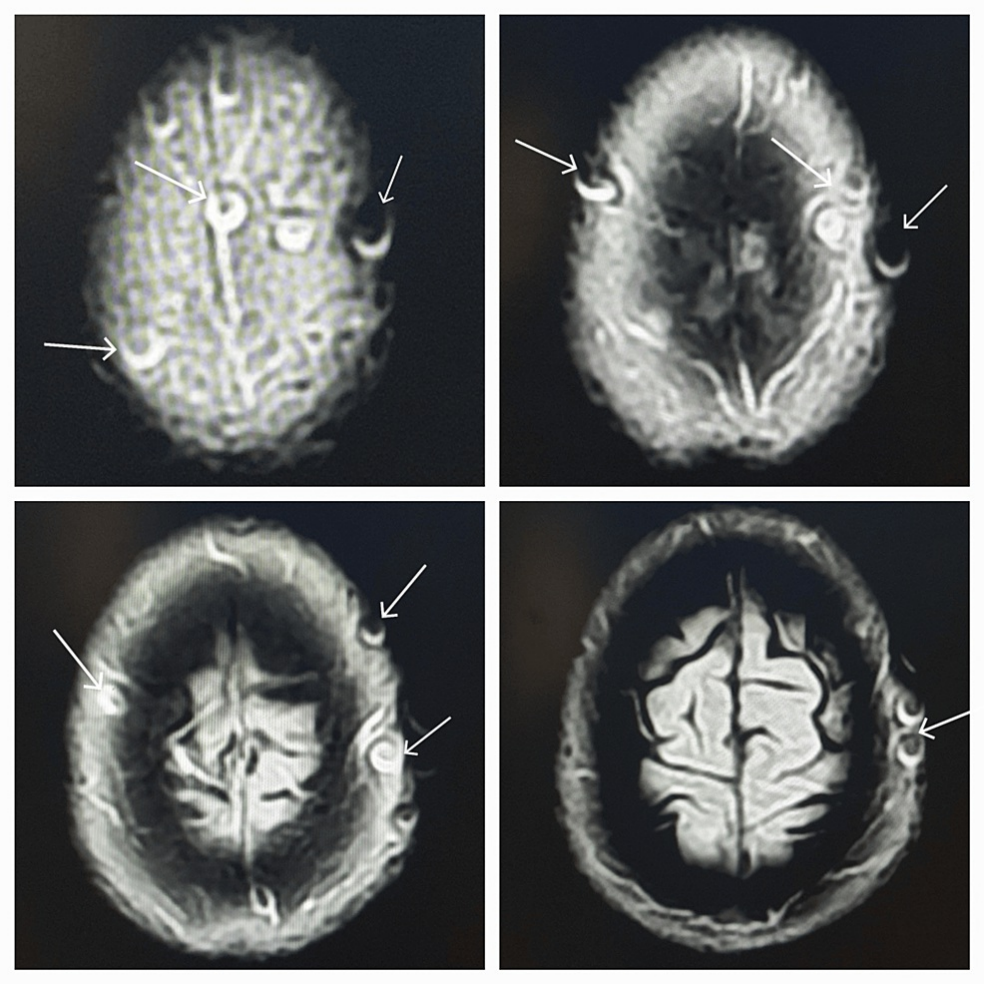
The artifacts are seen with central low and peripheral high signals within the scalp on the axial FLAIR sequence FLAIR: fluid attenuation inversion recovery
